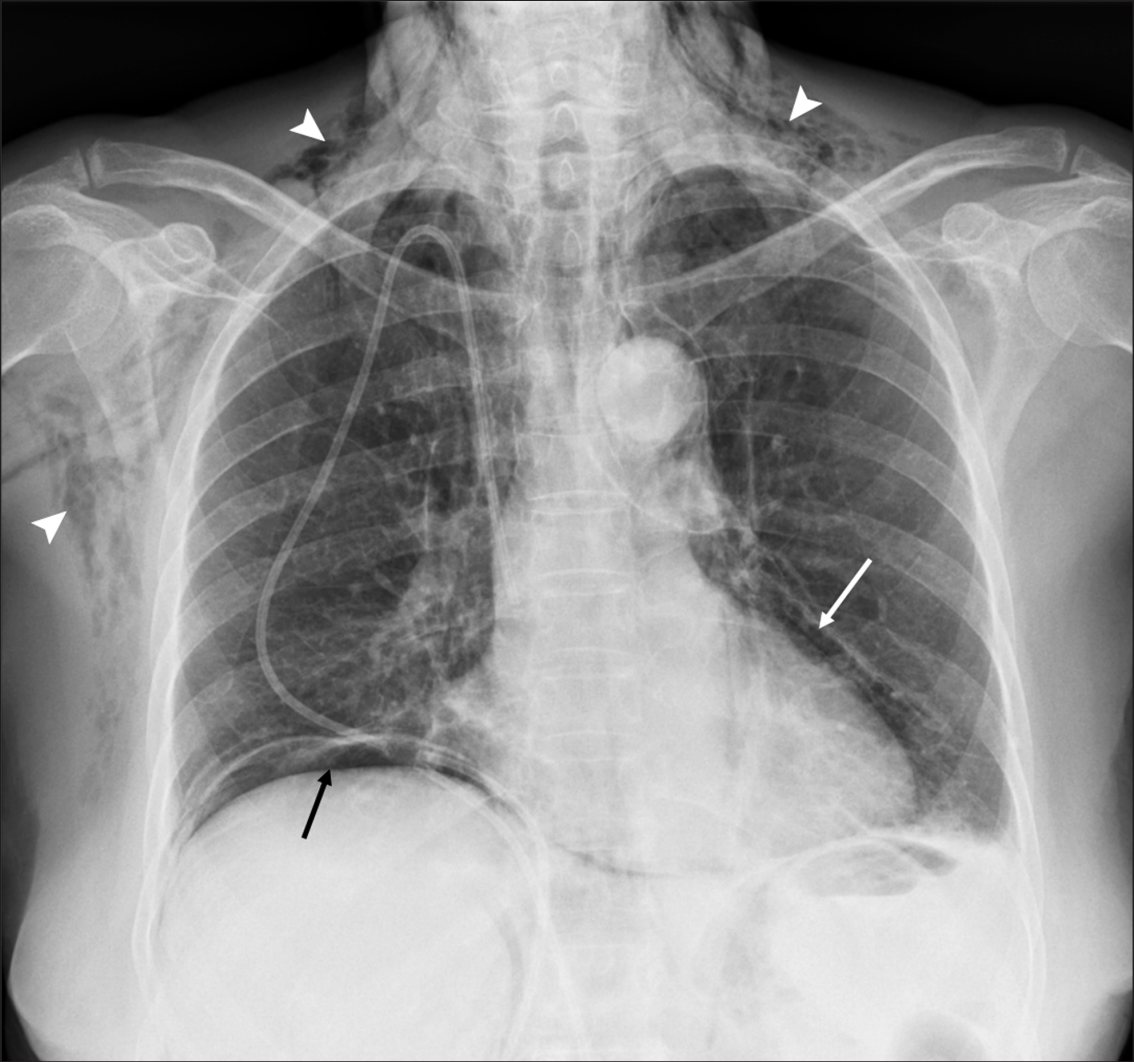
A Computed Tomography (CT) (*Figure 2*) was then requested, revealing extensive pneumatosis of the right and transverse colon (red arrows), pneumoperitoneum (asterisks), pneumomediastinum (white arrows), and subcutaneous emphysema (arrowheads). No evidence of gastrointestinal perforation was found on CT. The mesenteric vessels were patent and indirect signs of bowel ischemia, such as ascites or portal pneumatosis, were also absent.
